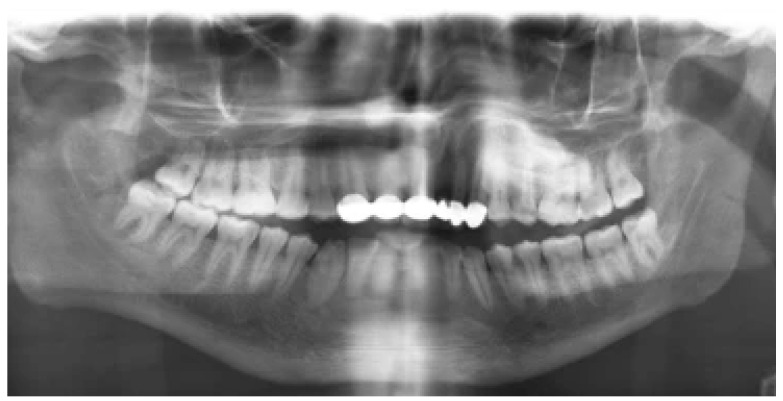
A panoramic radiograph. The symphyseal–parasymphyseal fracture is obscured by the superimposition of cervical vertebrae, and the condyle fracture is obscured by a partial image due to improper patient positioning. Published with permission from [28]. Copyright 2013, Elsevier.
